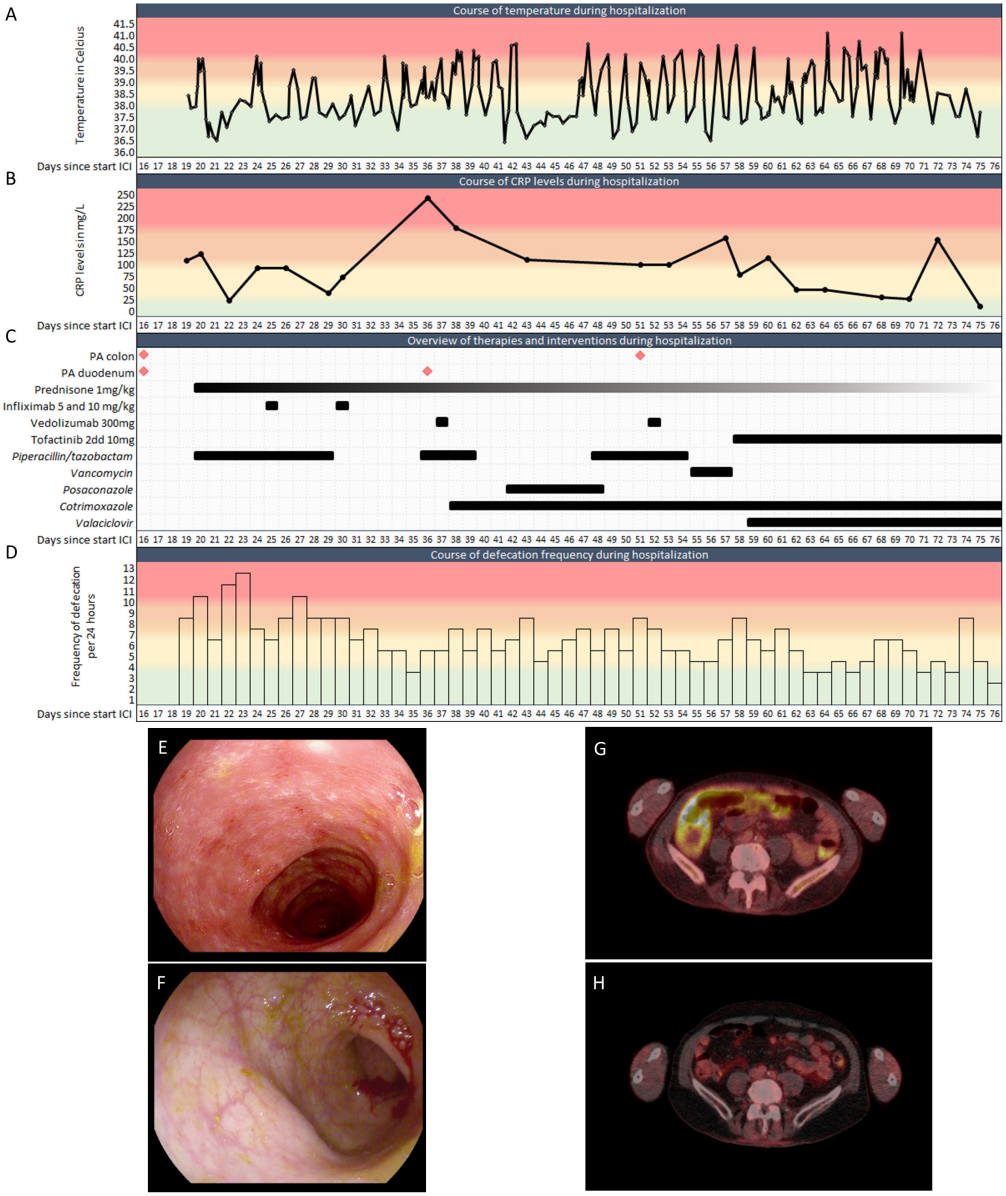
Clinical features of the patient during hospitalization. (A). Temperature during hospitalization (Celsius). (B). CRP levels during hospitalization. (C). Biopsies, anti-inflammatory drugs and antimicrobials during hospitalization. (D). Defecation frequency during hospitalization. (E, F). Representative images of sigmoidoscopies at (E) day 16 and (F) day 51. (G). PET-CT at day 48 with FDG-uptake in the colon. (H). PET-CT 3 months after discharge showing physiological FDG-uptake, demonstrating the absence of colitis after tofacitinib. PA: Pathological assessment; CRP: C-reactive protein; ICI: Immune checkpoint inhibitor. Colors indicate severity from green to red.
